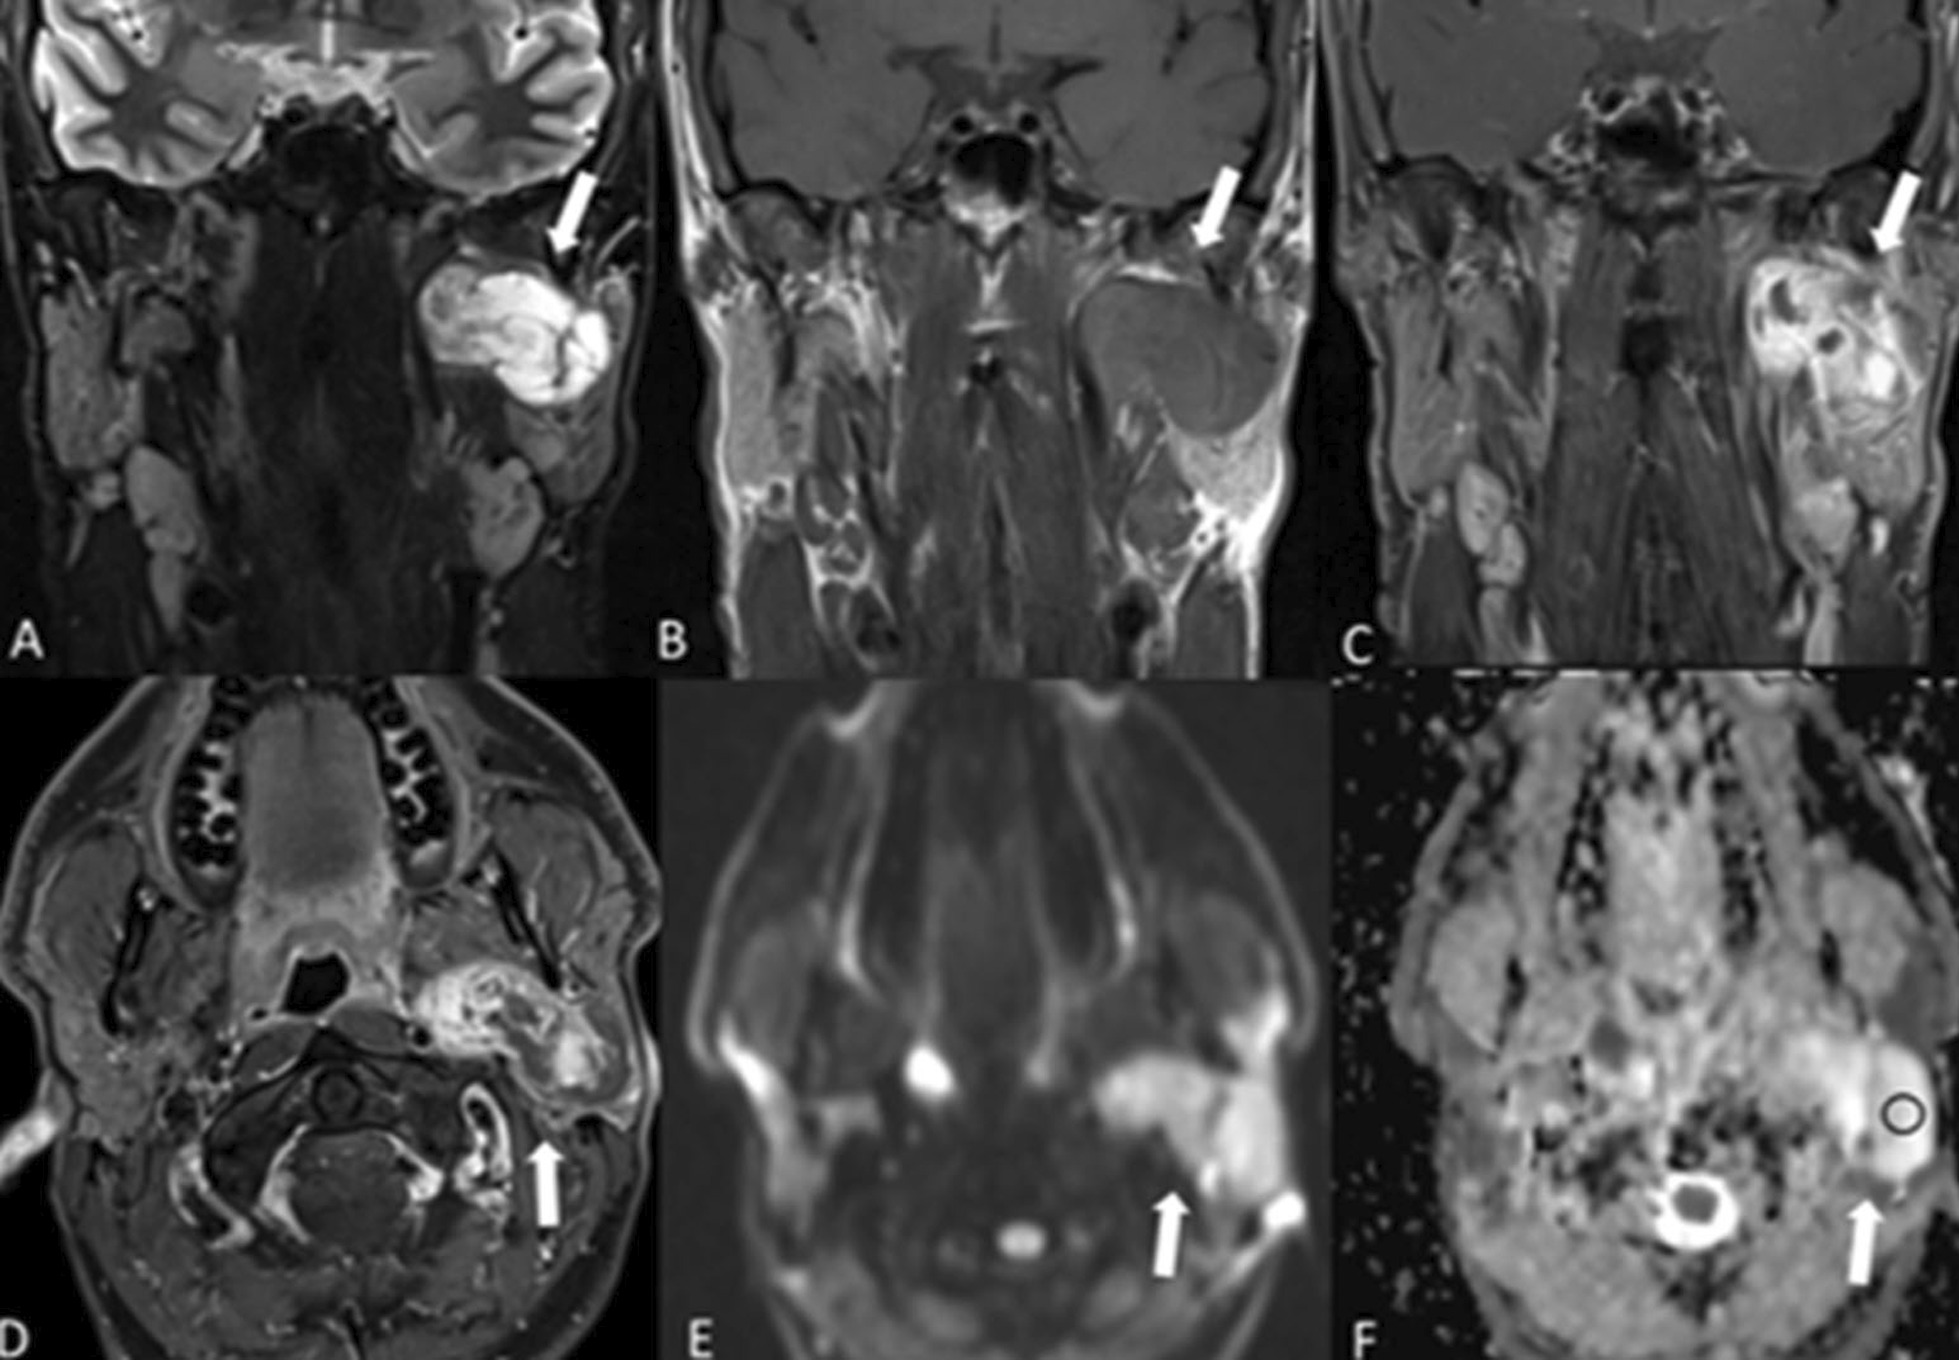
Pleomorphic adenoma. A 39-year-old male presenting with a painless preauricular swelling on the left side, which was proven to be a pleomorphic adenoma. Coronal T2-w MRI (A) shows a predominantly hyperintense lesion of the left parotid gland (arrow), which is hypointense on T1-weighted image (B). The lesion lies in both the deep and the superficial parotid with an hourglass configuration. The coronal (C) and axial (D) images after injection of intravenous gadolinium with fat saturation shows heterogeneous enhancement of the lesion. The signal intensity is high in the diffusion-weighted image (E) and it remains high in the ADC maps (F) with ADC values (o) of 2300 ± 270 × 10^−6^ mm^2^/s and this is helpful for identifying pleomorphic adenoma
